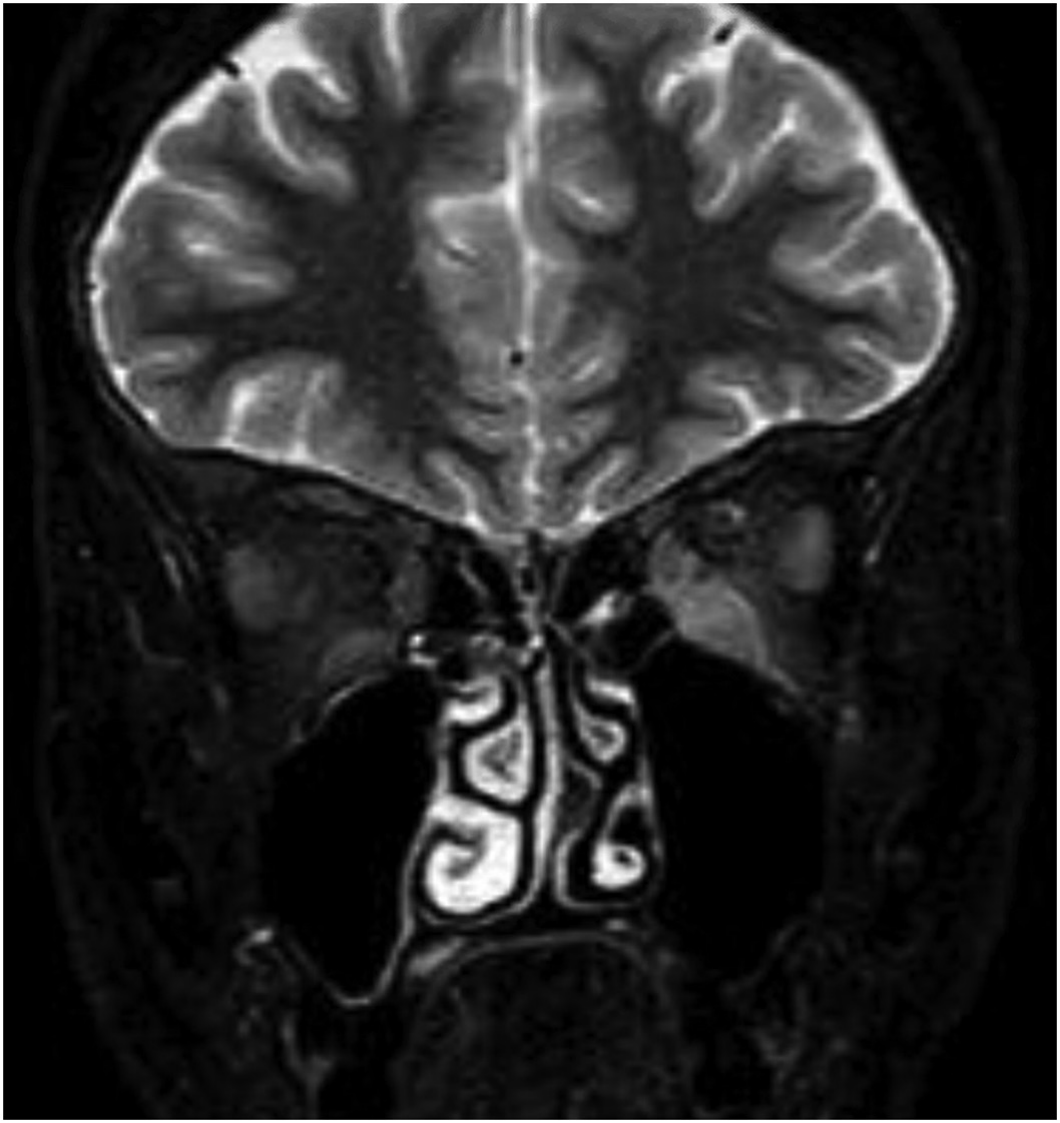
Initial MRI orbits STIR sequence coronal view; there is increased thickness of the inferior and medial rectus muscles bilaterally, particularly the right inferior rectus.
